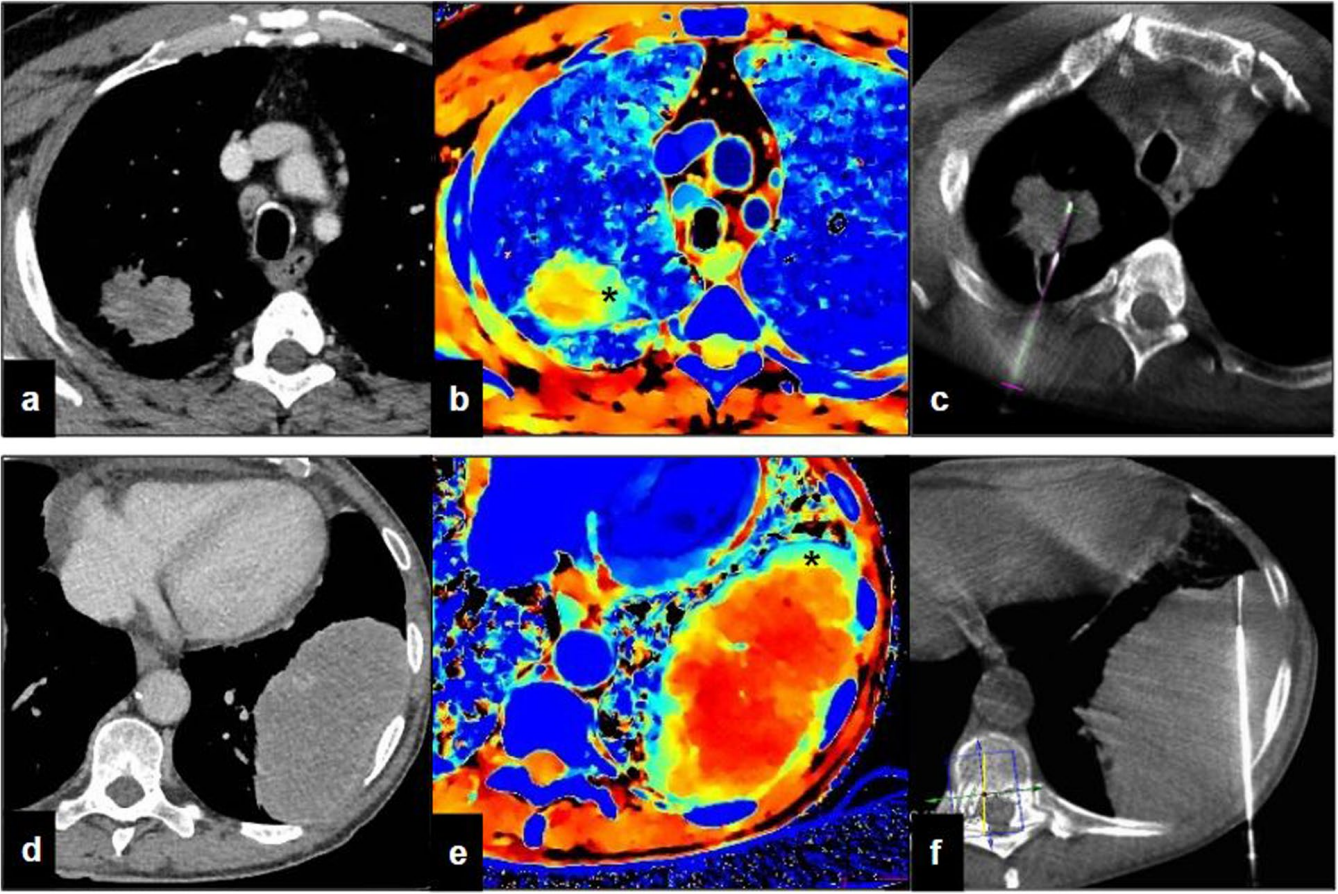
Percutaneous lung biopsy. a Axial routine diagnostic image in a patient with solid lung lesion at the upper right lobe. b Effective atomic number-based reconstruction at the same level: the lesion is characterised by inhomogeneous intralesional atomic number with a central area showing a reduced atomic number (yellow–red) and a medial eccentric area showing a higher atomic number (light blue, asterisk). c Intraprocedural cone beam computed tomography (CBCT) shows that the needle has been located in the area with the highest atomic number. d Axial routine diagnostic image in a patient with solid lung lesion at the lower left lobe. e Effective atomic number-based reconstruction at the same level: the lesion is characterised by inhomogeneous intralesional atomic number with a central area showing a reduced atomic number (yellow–red) and an external circular area showing a higher atomic number (light blue, asterisk). f Intraprocedural CBCT shows that the needle has been located in the area with the highest atomic number
